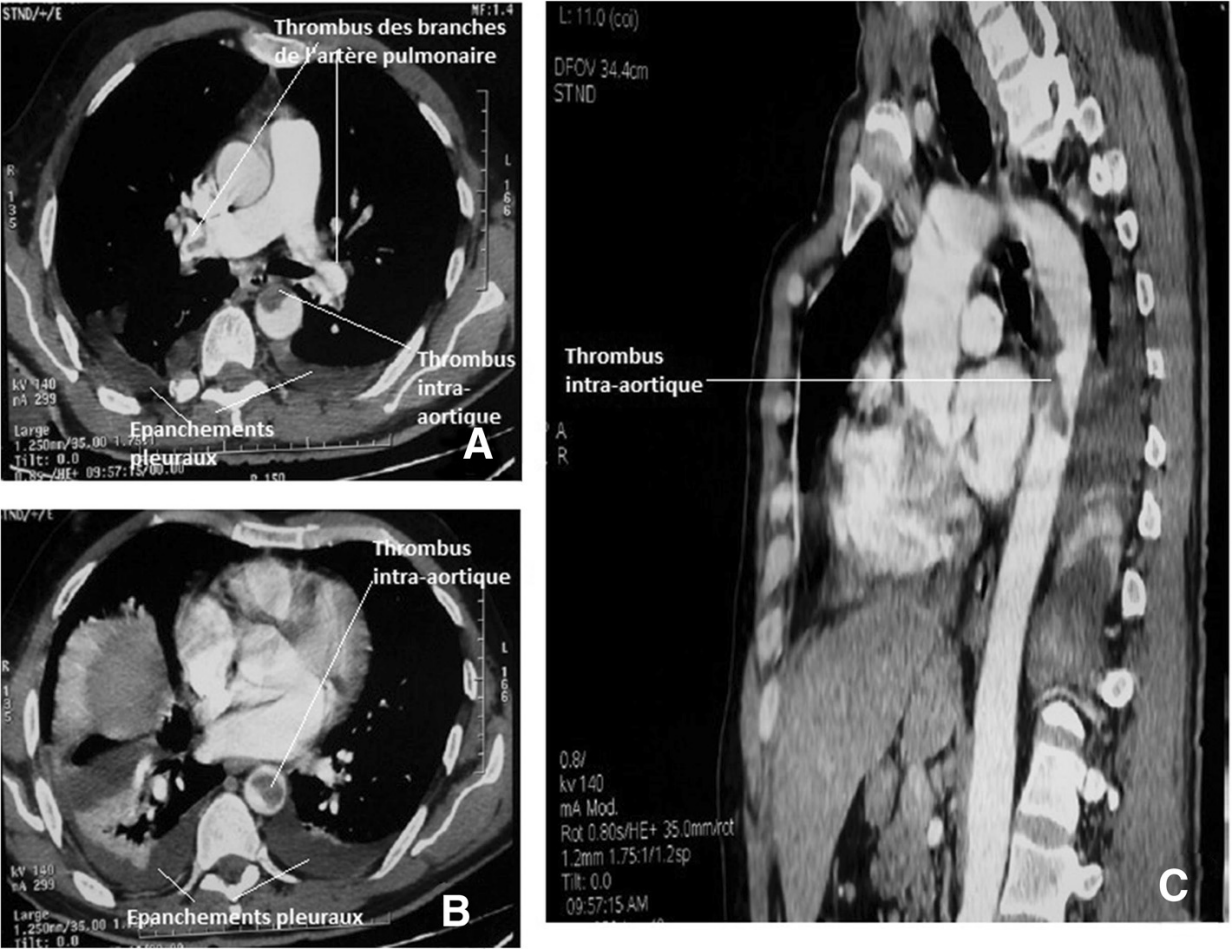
CT angiography showing a thrombus in the pulmonary artery branches. Axial section A; bilateral pleural effusions, Axial A and B; persistence of intra aortic thrombus. Axial section A and B and sagittal reconstruction C.
